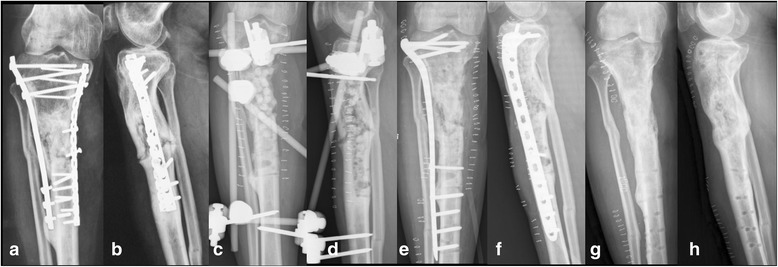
A 41-year-old male suffered from osteomyelitis of the right proximal tibia. Radiographs on admission showed loose and broken screws (a-b). The hardware was removed and a thorough debridement was performed. Partial segmental bone defect occurred after debridement. The bone defect was filled with cement beads, and the tibia was stabilized with a standard external fixator (c-d). Eighty days later, the cement beads were removed; cancellous autografts were placed within the bone defect and the external fixator was exchanged with internal fixation (e-f). The bone was healed at 11 months after the bone grafting; radiographs at 2 years follow-up showed bone union (g-h)
